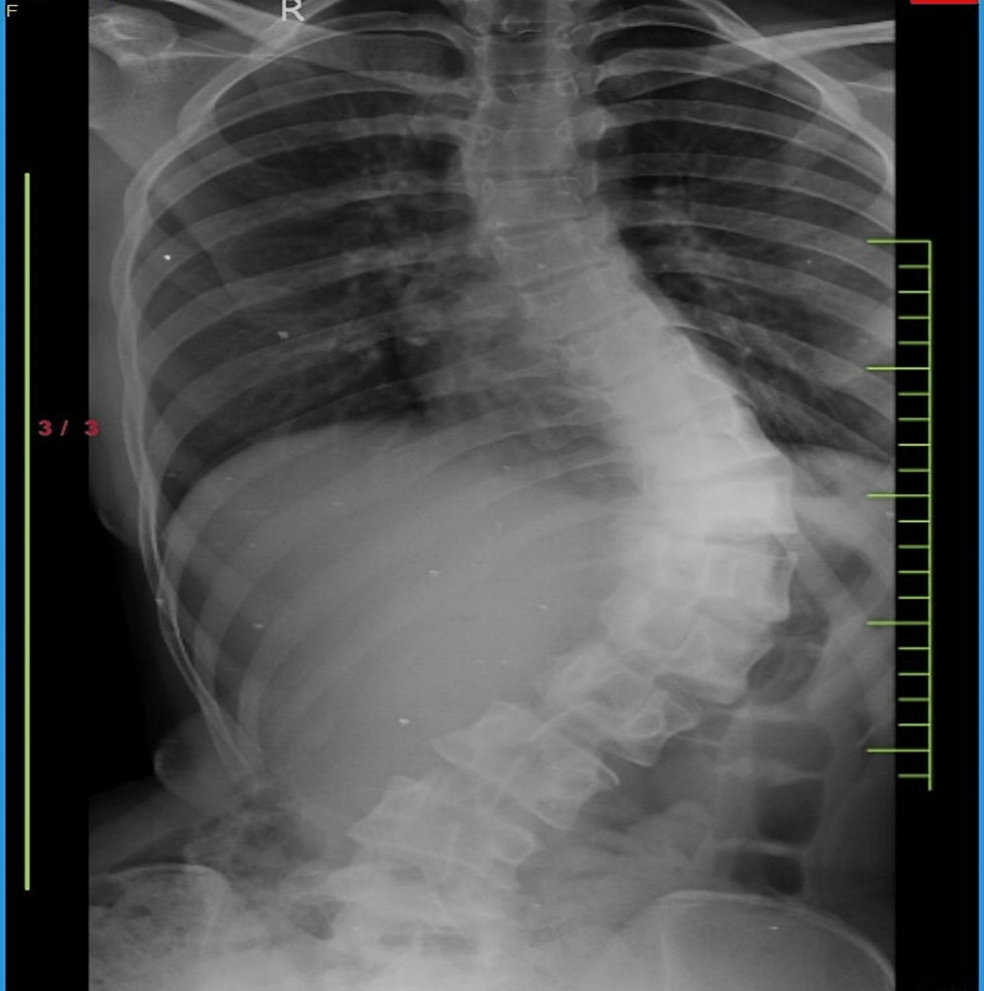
Preoperative X-ray showing the degree of scoliosis.
